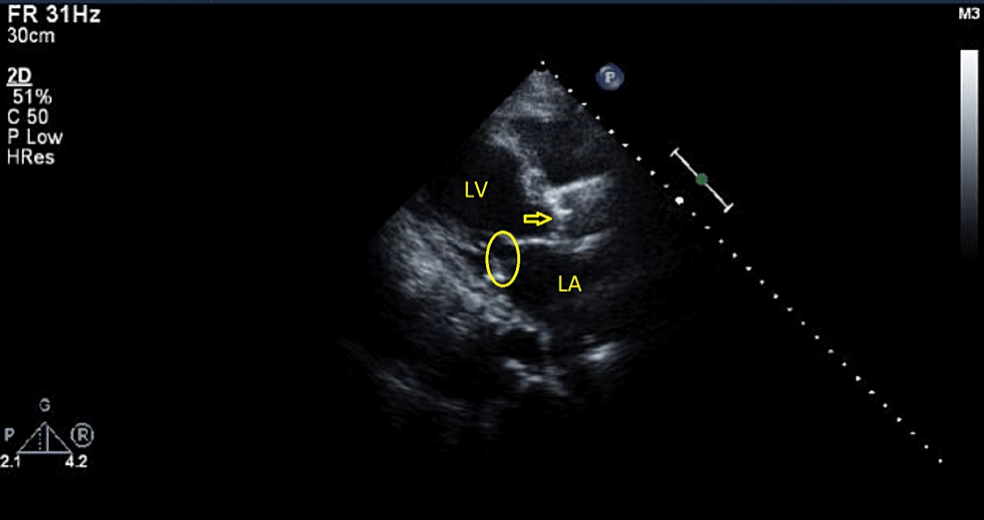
2D ECHO image showing the opening of MV and closed AV with thickening of MV leaflets 2D ECHO: two-dimensional echocardiography; MV: mitral valve; AV: aortic valve; LA: left atrium; LV: left ventricle.
